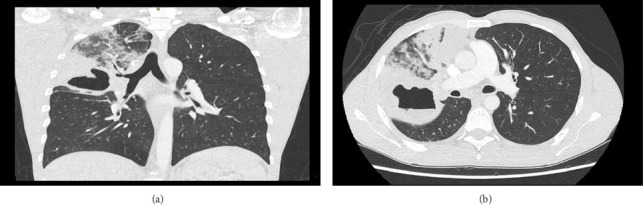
(a) Coronal section and (b) axial section of chest CT scan reveal extensive multifocal consolidation in the right upper lobe, including a large thick-walled cavity in the posterior segment, measuring approximately 6.8 × 7.6 × 6.1 cm. Additional features include clustered and tree-in-bud nodules, along with patchy foci of consolidation in the right middle lobe, lingula, and left lower lobe.
